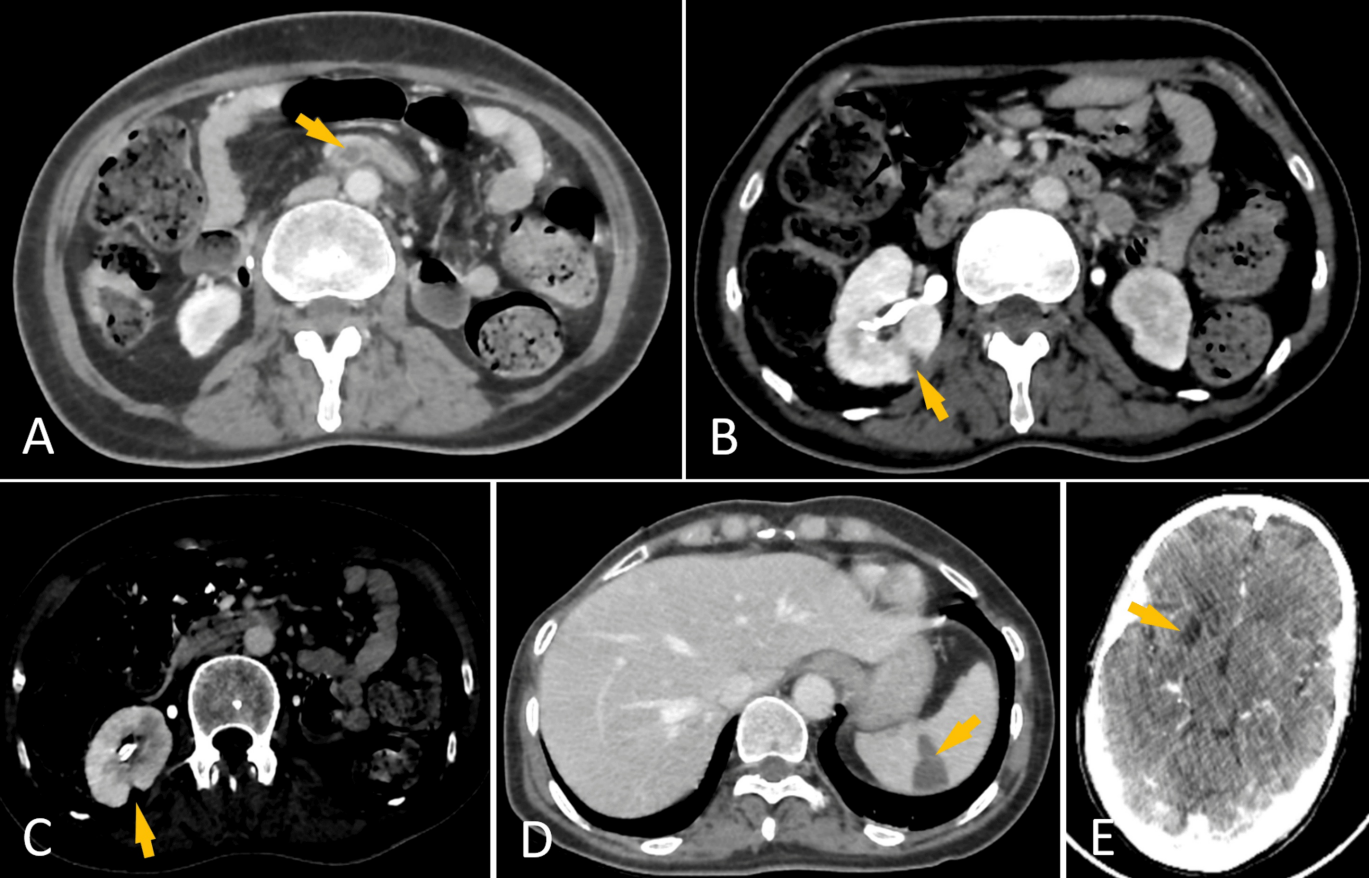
A: Axial abdominal CT scan: Luminal defect of the superior mesenteric artery, consistent with thromboembolism (Arrow). B: Axial abdominal CT scan: Wedge-shaped hypodense area of the right kidney, indicating renal infarction (Arrow). C: Axial abdominal CT scan: Wedge-shaped hypodense area of the right kidney, indicating renal infarction (Arrow). D: Axial abdominal CT scan: Hypodense, wedge-shaped area in the spleen, consistent with splenic infarction (Arrow). E: Axial brain CT scan: Right caudo-lenticulo-capsular ischemic lesion (Arrow).
